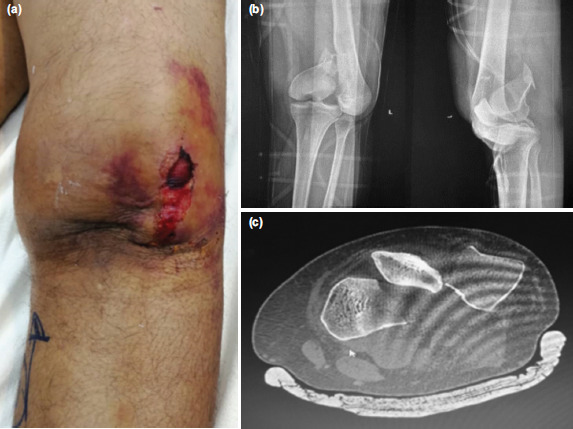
(a) Physical examination revealed a swollen left knee with a palpable gap at the anterior knee, patella cannot be felt. (b) Plain radiograph of left knee showing patella was rotated and incarcerated in medial condyle femur fracture site (c) Axial view of left knee CT, showing rotational dislocation of the patella.
